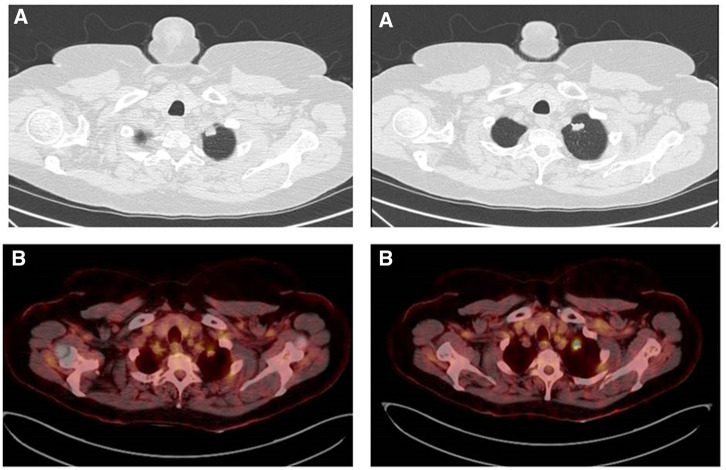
(A) Computed tomography showing a solid nodule in the left upper lobe. (B) Positron emission tomography showing a lung nodule with metabolic activity.
